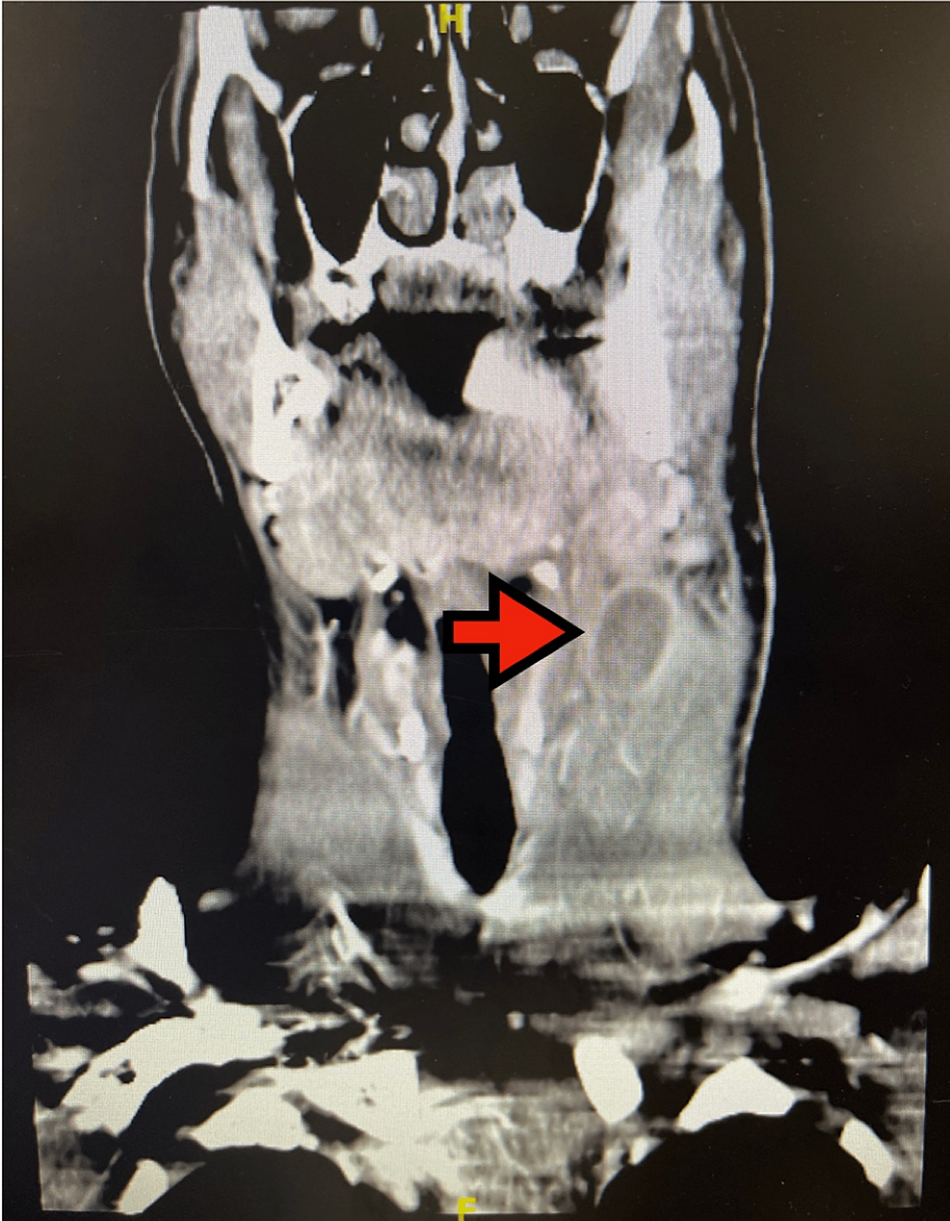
Frontal view CT scan. Red arrow demonstrates left neck mass, likely lymphatic in origin. CT: computed tomography
